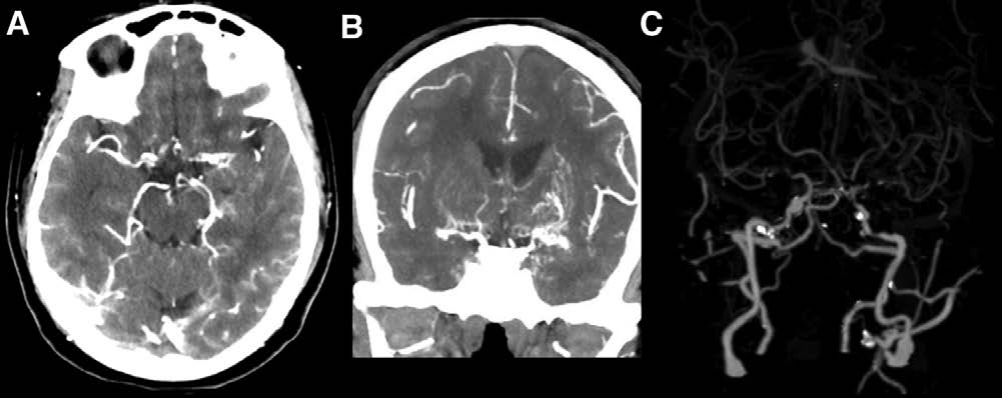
(a) Axial CTA image showing stenosis of the distal internal carotid arteries and M1 segments. (b) Coronal CTA image demonstrating the bilateral thalamic collaterals. (c) MIP CTA image show stenosis of the distal internal carotid arteries. CTA = computed tomography angiography, MIP = maximum intensity projection.
